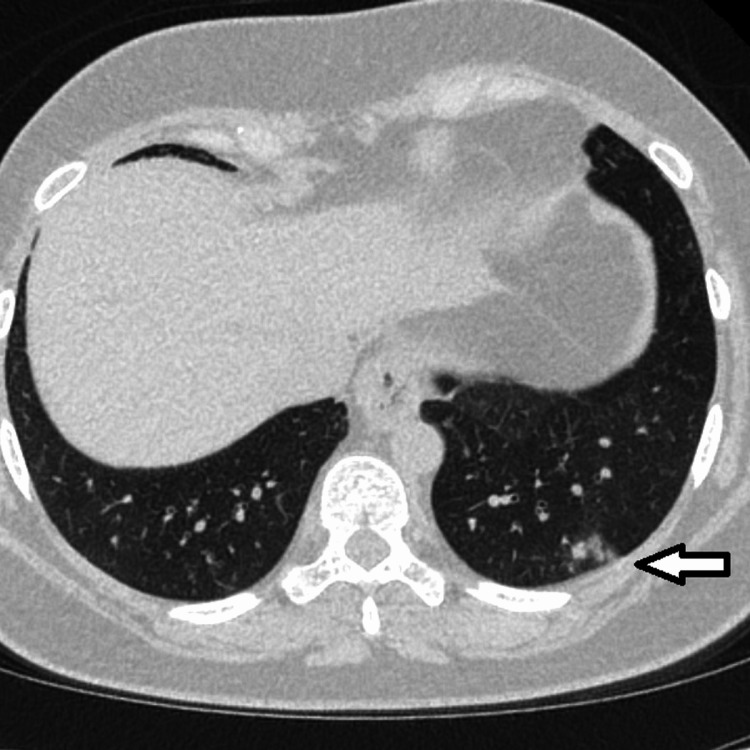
CT of the chest. Lung window. Lung lower lobes. Left lower lobe nodules (arrow).
